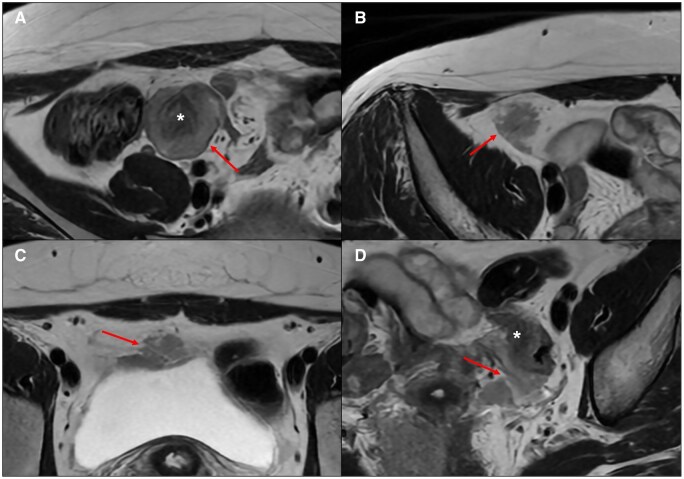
Decidualized DPE at multiple pelvic sites. Axial T2WI images demonstrate additional solid components with similar MRI characteristics at multiple pelvic locations: involving the terminal ileum (A, arrow), adjacent to a bowel endometriosis nodule (star in A); along the right round ligament (B, arrow); in the prevesical space (C, arrow); and between the left parametrium and the mid-sigmoid colon (D, arrow), site of another bowel endometriosis nodule (star in D).
